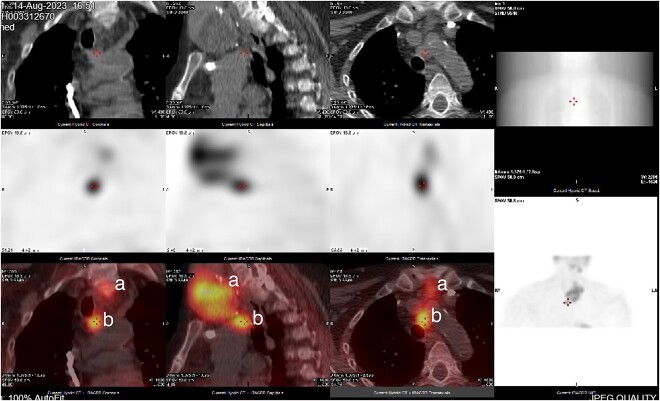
CT neck and sestamibi scan of coronal, sagittal, and axial view of superior mediastinum with mediastinal ectopic parathyroid nodule. (a) Thy3A thyroid nodule; (b) mediastinal parathyroid nodule.
